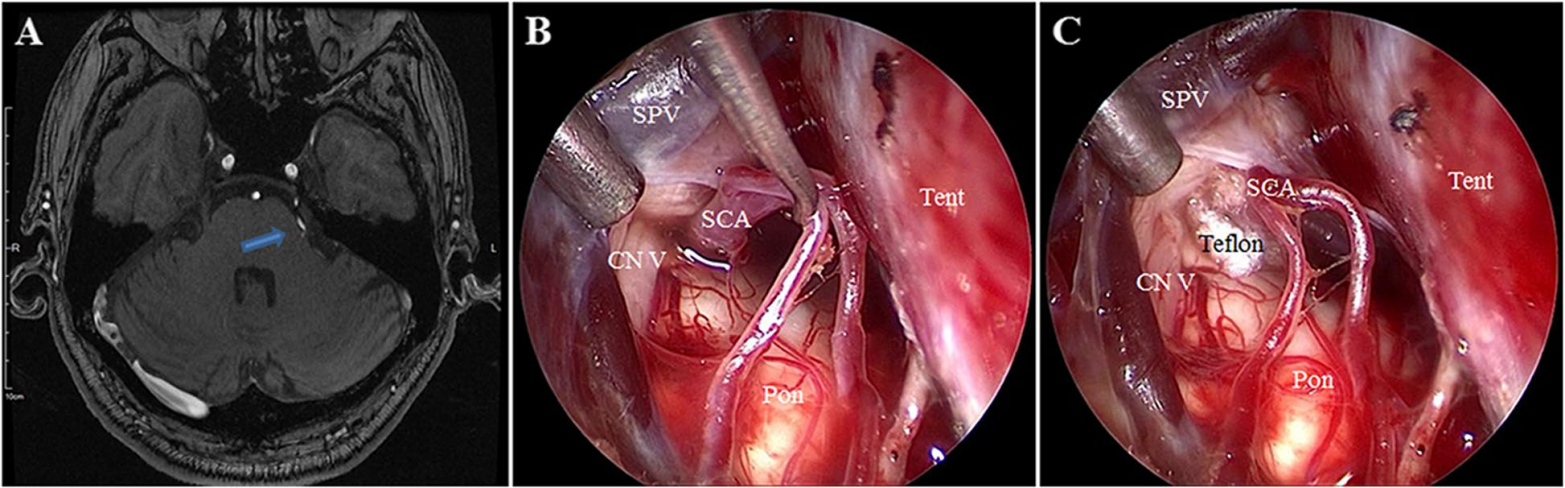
Preoperative and intraoperative findings in case 1. A Preoperative head magnetic resonance imaging of the patient in case 1. B Under the endoscopic view, the trigeminal nerve was compressed into an angle by the SCA in the root entry zone. C The Teflon pads were placed between the trigeminal nerve and the SCA under the endoscopic view. The blue arrow indicated the trigeminal nerve compression by the offending vessel. SPV, superior petrosal vein; SCA, superior cerebellar artery; CN V, trigeminal nerve; Ten, tentorium; Pon, Pons
